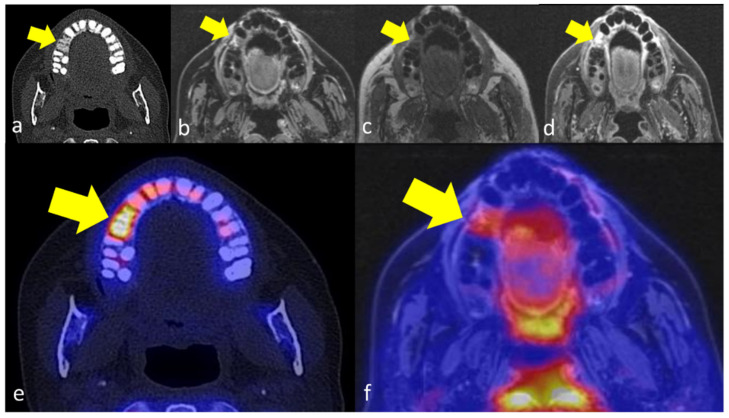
49 years old female patient with secondary chronic OMJ in region 15 of the maxilla (yellow arrows). The OM affected jawbone shows a diffuse trabecular sclerosis in CT (a), an edema in T2-weighted images (b) and corresponding T1-weighted images (c) with increased contrast enhancement after gadolinium application (d). The corresponding PET images show a markedly increased [18F]fluoride uptake in the affected jawbone (e) and an increased [18F]FDG-uptake (f).
